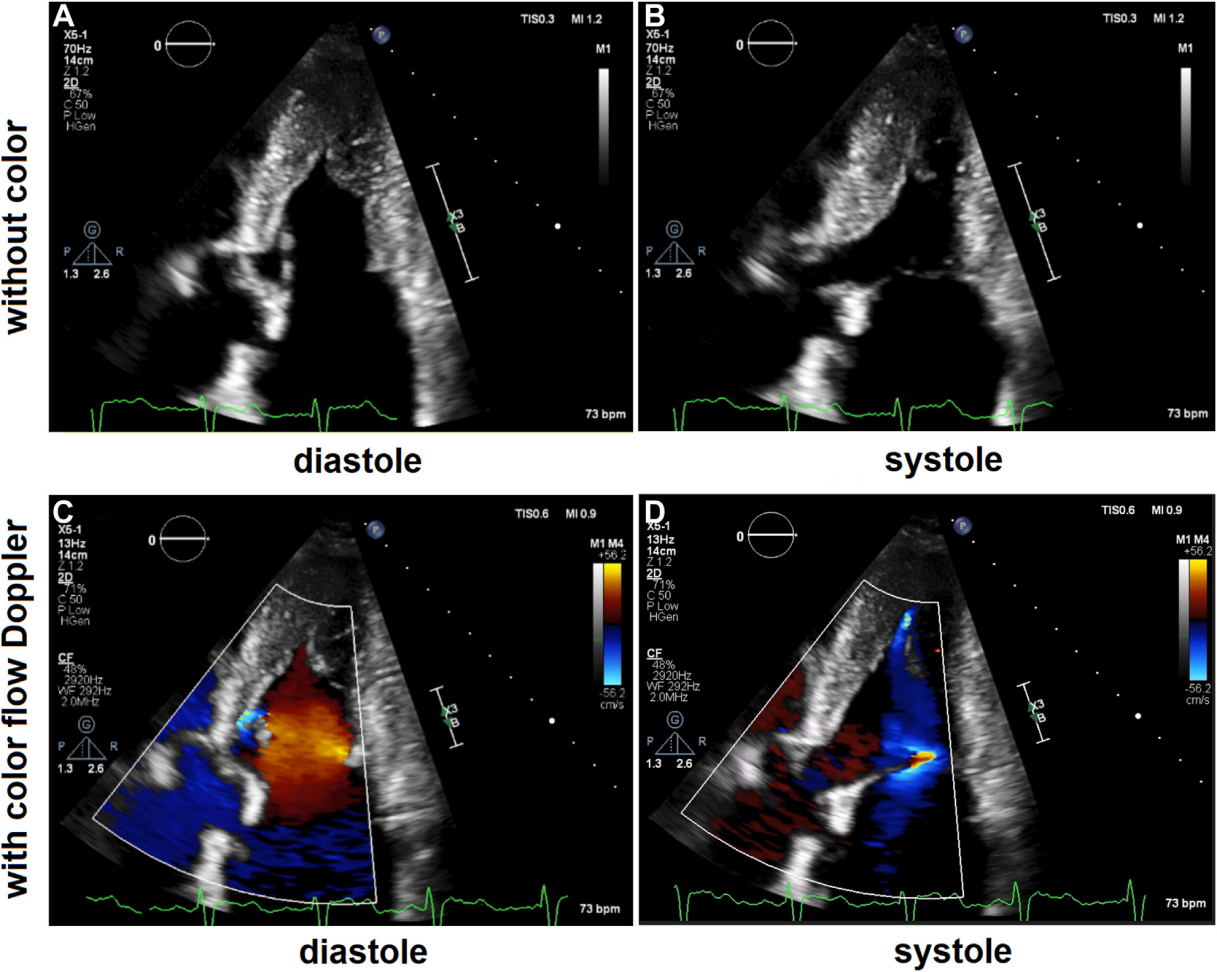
Two-dimensional TTE, apical 5-chamber view without (*top*) and with (*bottom*) color-flow Doppler in diastole (*left*) and systole (*right*), demonstrates AHCM with a slit-like LV cavity toward the cardiac apex.
